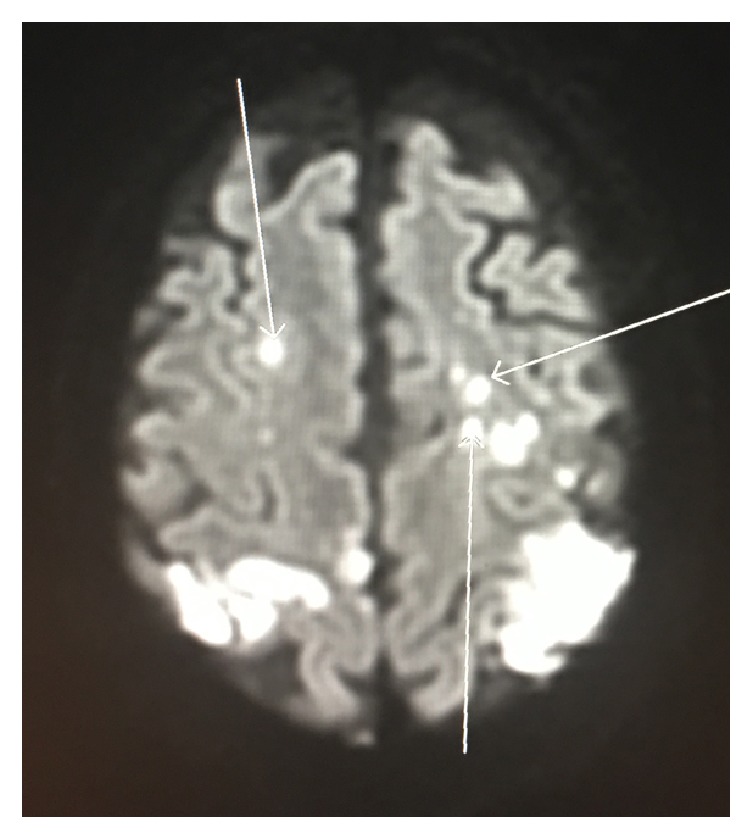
Brain MRI showing multiple septic emboli.
